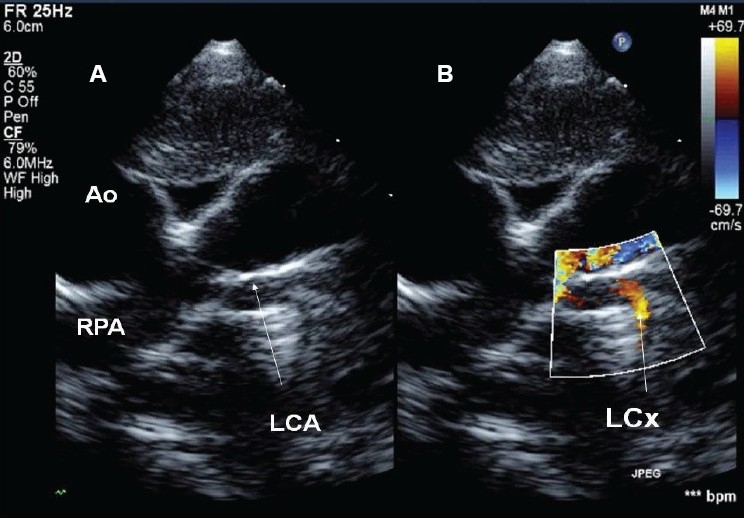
(A, B): These echocardiograms have been obtained from patient #3. (A) Left coronary artery arising from right pulmonary artery (B) retrograde flow from coronary to pulmonary artery. Ao: aorta, LCA: left coronary artery, LCx: Left circumflex artery, RPA: right pulmonary artery
